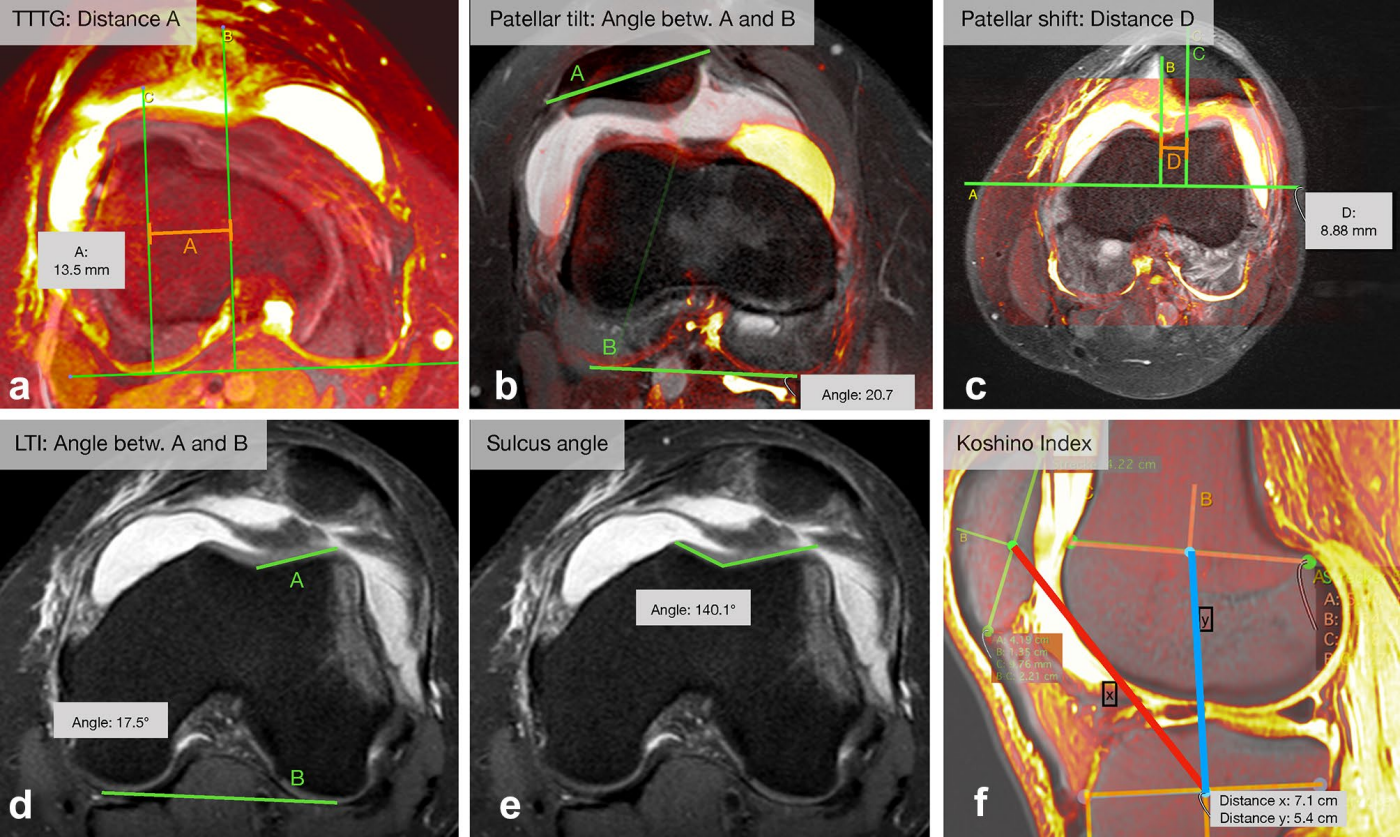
Overview of MRI parameters measured with Osirix. a Overlapped axial slices to measure TT-TG (tibial tubercle-trochlear groove), b patellar tilt c patellar shift. d axial images to measure LTI (lateral trochlear index), e the sulcus angle. f sagittal image to measure the Koshino index
